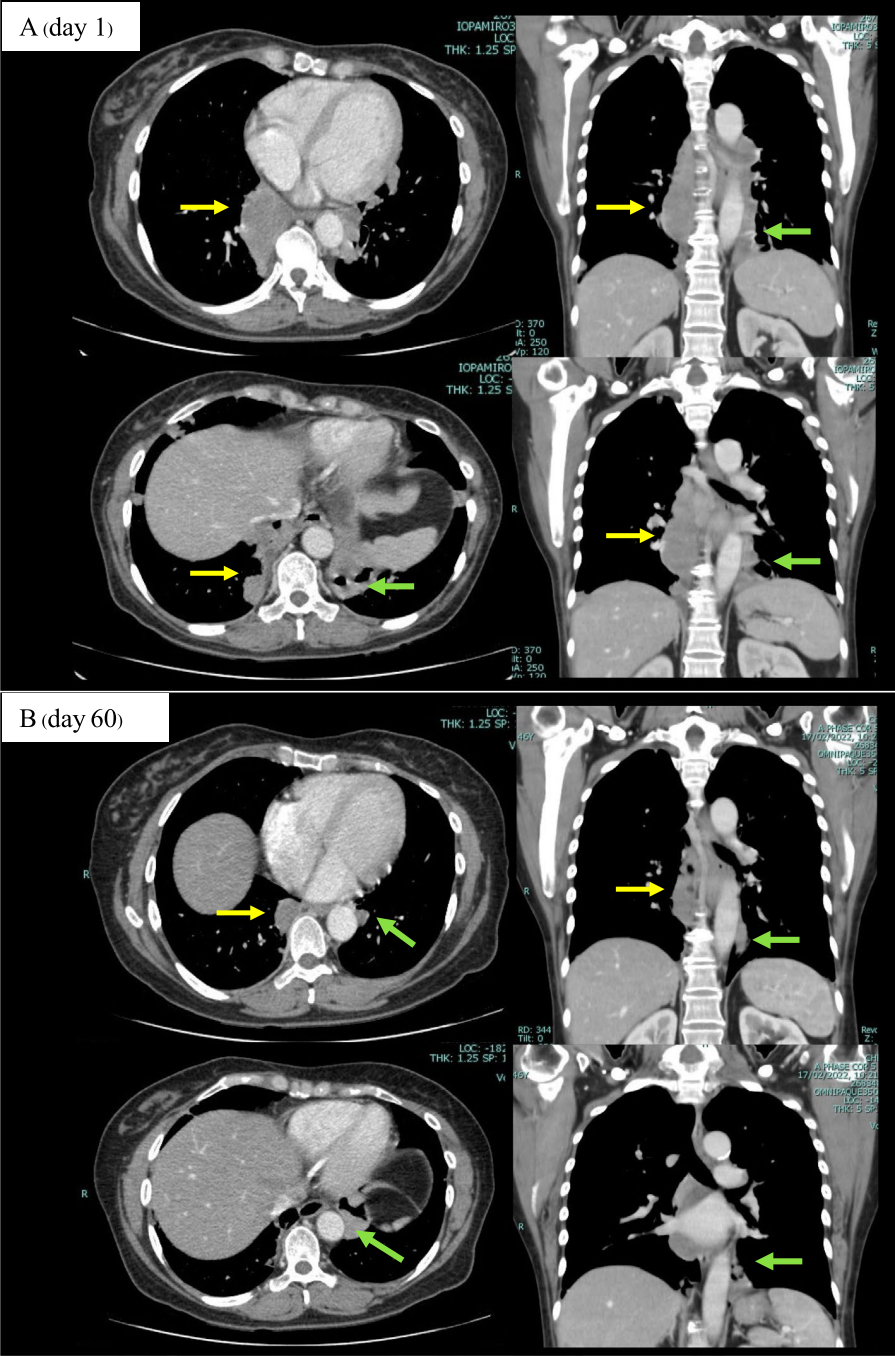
Computed tomography (CT) scan of the chest in transverse section on the left and coronal section on the right showed an enhancing soft tissue mass-like lesion (3.8 × 6.6 × 9.3 cm) at the right paravertebral region T5-T10 level (yellow arrows) and 3.1 × 4.6 cm thick-walled cavity lesion at the left lower lung (green arrows) (A-day 1) that decreased in size to 3.2 × 2.4 × 7.3 cm of right paravertebral soft tissue mass (yellow arrows) and 2.3 × 2.9 cm of left lower lung lesion (green arrows) after 2 months of initiating treatment (B-day 60)
